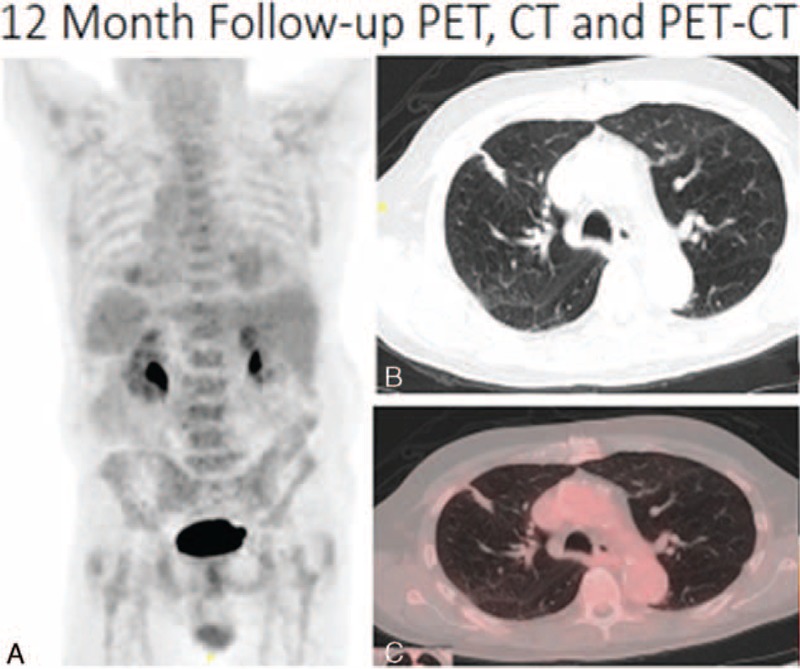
Reveals PET, CT, and PET-CT findings at 12-month follow-up. A (whole body PET) does not demonstrate evidence FDG uptake in the cryoablation zone where preablation was evidence of radiotracer activity. B and C reveal residual scar-like opacity in the right upper lobe cryoablation zone without interval increase in CT attenuation of the cryoablation zone.
